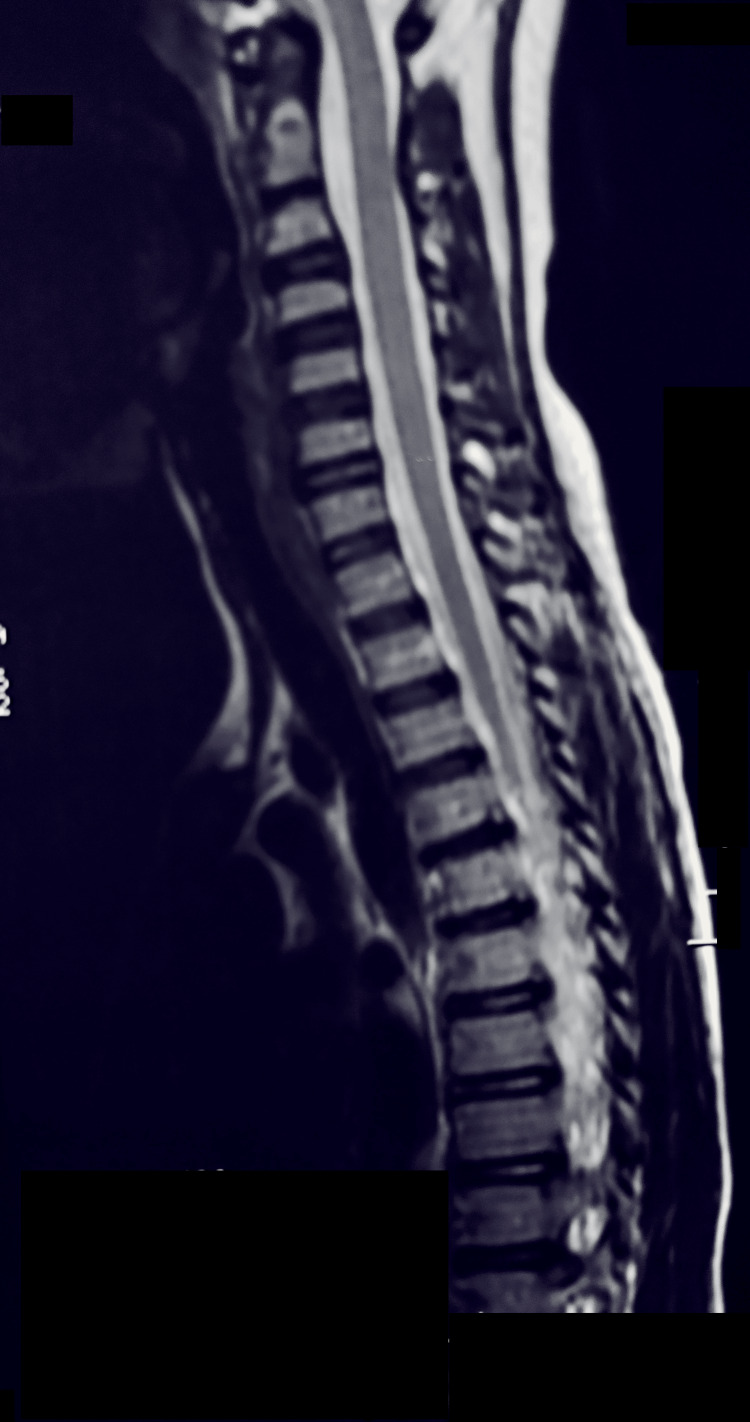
Sagittal MRI of the cervical and upper thoracic spine showing normal vertebral alignment, intact intervertebral discs, and no evidence of spinal cord compression or osseous lesions MRI: magnetic resonance imaging
